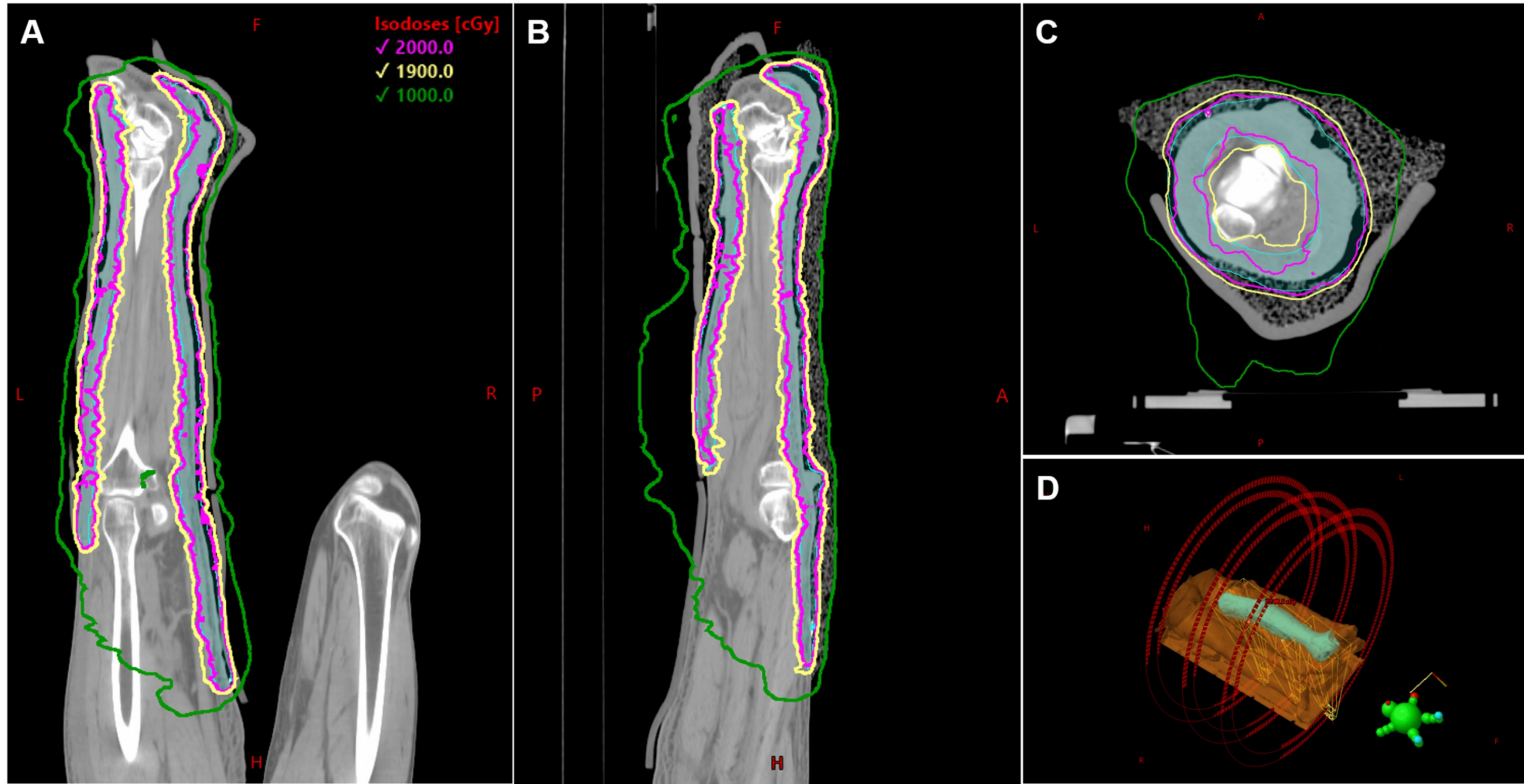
Isodose distribution in the coronal (A), sagittal (B), and axial (C) planes of the CT planning dataset with a prescription (Rx) of 20 Gy in five fractions. The PTV is shown in cyan, and isodose lines of 2000 cGy (Rx dose), 1900 cGy (90% of Rx), and 1000 cGy (50% of Rx) are shown in pink, yellow, and green, respectively. (D) shows a 3D rendering of the body and Vac-Lok (orange) and PTV with the treatment arcs (red curves) split into three isocenters across the superior-inferior extent of the PTV. Avoidance sectors were used to avoid beam entry through the right leg. cGy: centigray; L: left; R: right; A: anterior; P: posterior; H: head; F: foot; PTV: planned target volume
